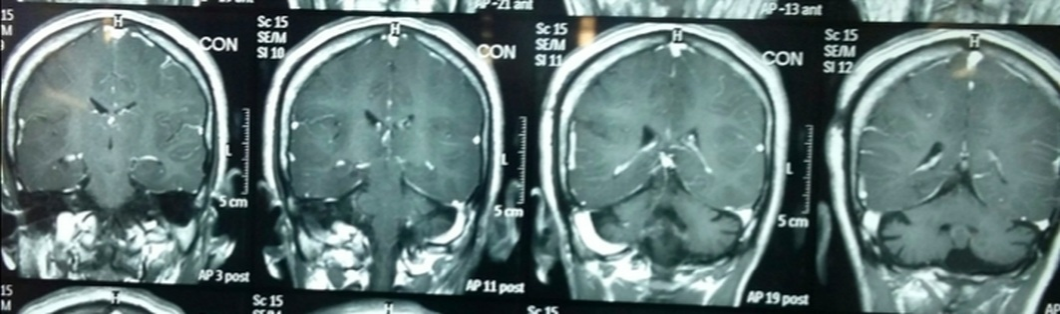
MRI Revealing Cortical Dysplasia and Multiple Hamartomas in the Medial Temporal Lobe, Thalamus, and Periventricular Region
